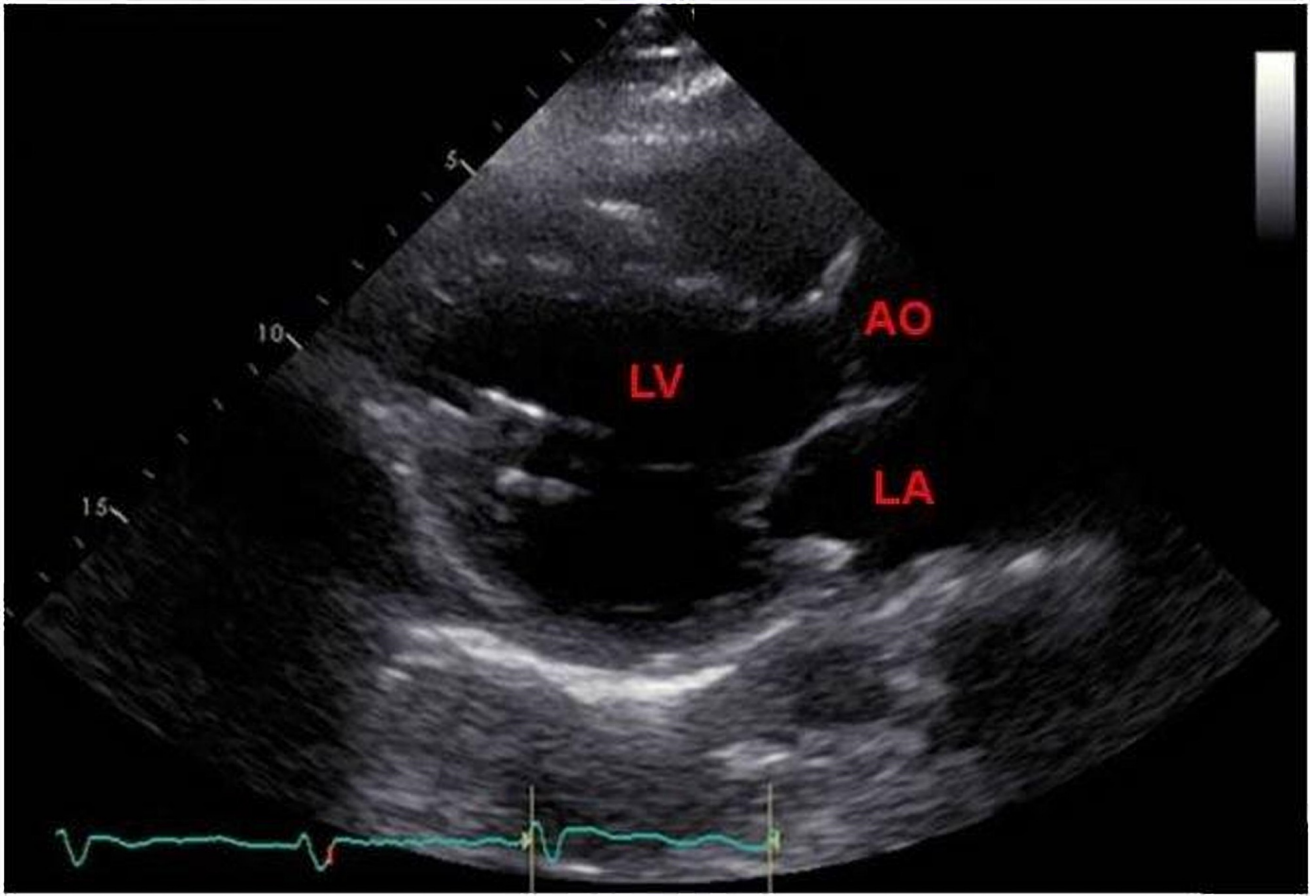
Follow-up transthoracic echocardiography showed the absence of microbubbles in the aorta and left ventricle LV: left ventricle; AO: aorta; LA: left atrium
